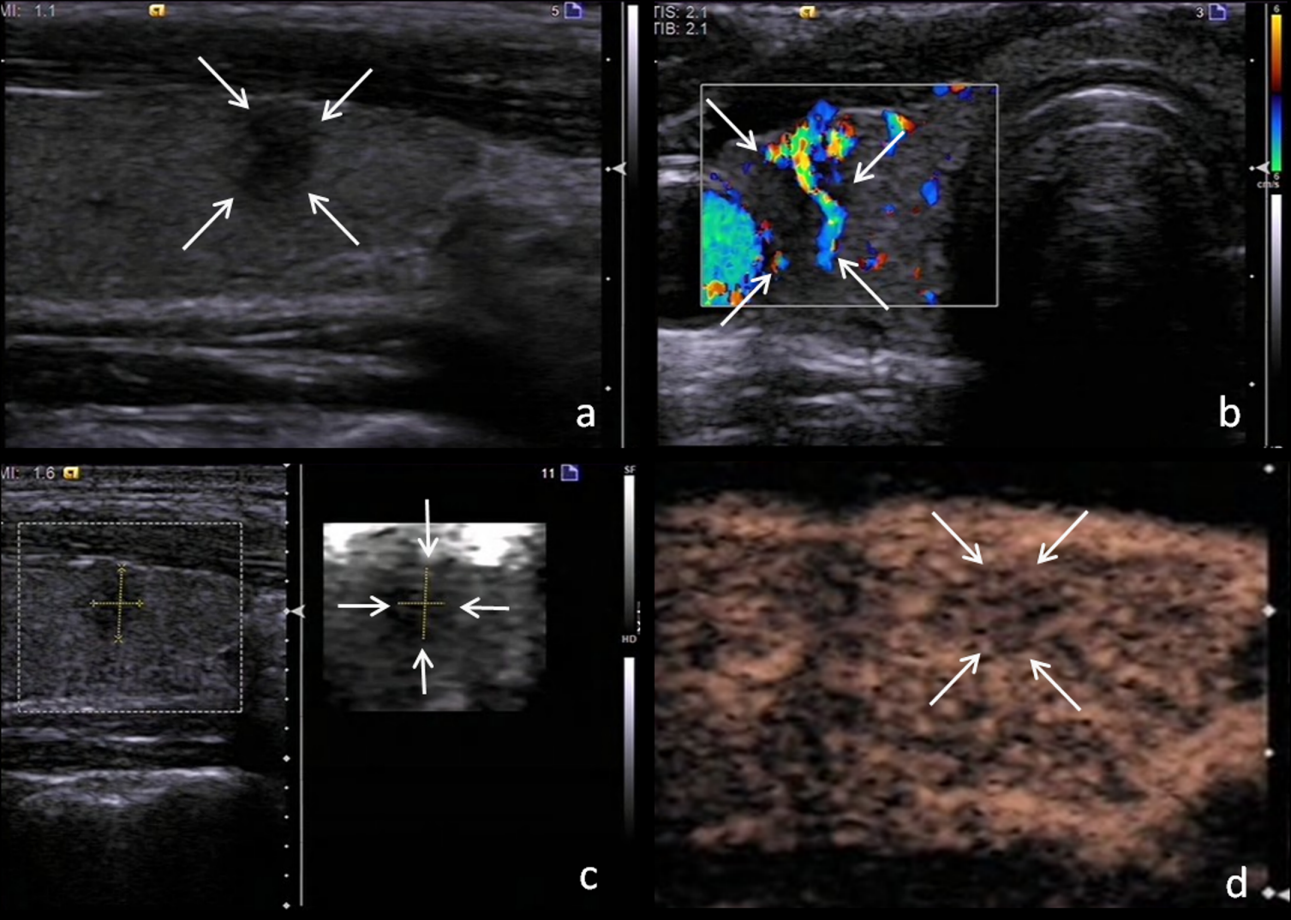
Ultrasonography images of a malignant thyroid nodule in the right lobe of a 44-year-old woman. The calculated predictive value with the logistic regression formula was 0.980. Surgical pathology proved a papillary thyroid carcinoma. (a) Conventional US image showing a thyroid nodule with irregular margin, ill-defined border, taller-than-wide shape, hypoechogenicity in the longitudinal section. (b) Color Doppler US image showing central vascularity in the transversal section. (c) SE image showing elastography score of 4 in the longitudinal section. (d) CEUS image showing a heterogeneous mass with hypo-enhancement pattern in the longitudinal section.
